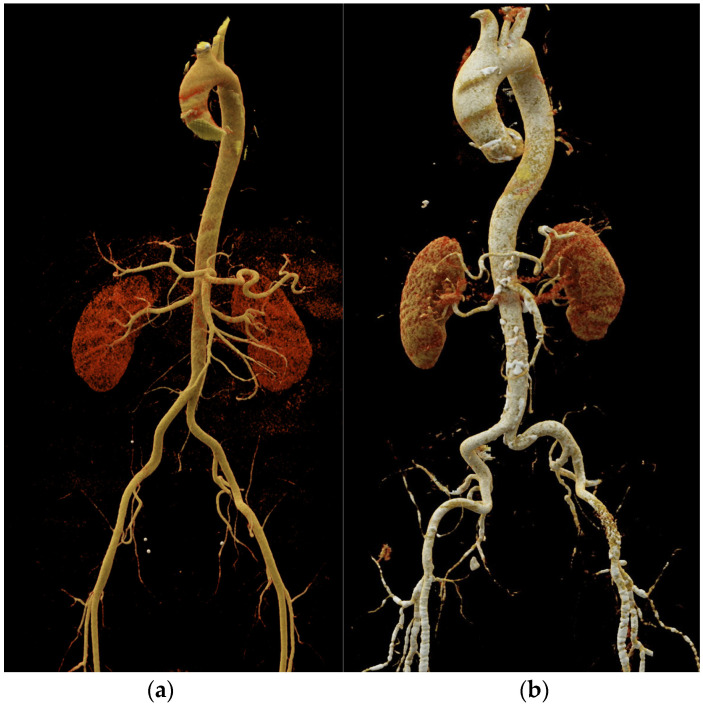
Volume rendering of a CT angiography in a 44-year-old male (a) and an 86-year-old male (b), with aging arteries become wider, tortuous, and elongated.
